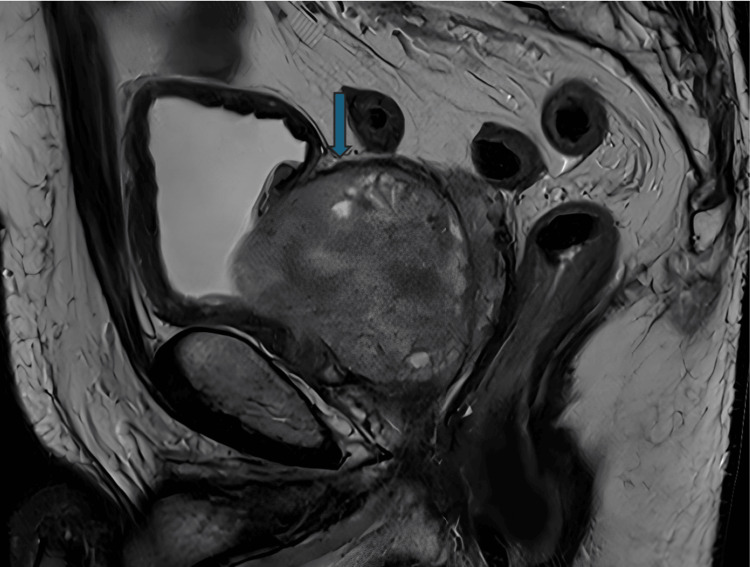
Sagittal view of the MRI demonstrating bi-lobar enlarged prostate (blue arrow)
